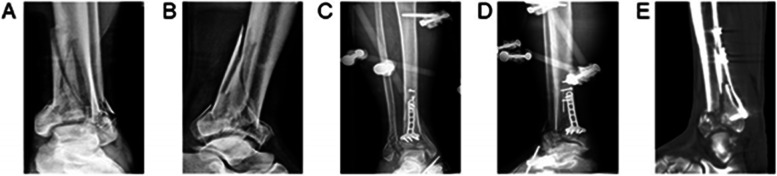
A 52-year-old man involved in a motor vehicle accident developed AO/OTA C3 pilon fracture. A & B) Preoperative anteroposterior and lateral radiographs showed the fibular fracture and distal tibial articular surface multi-fragment. C & D) The anteroposterior and lateral view of the ankle showed external fixation was used to maintain the length and axial alignment of the limb undergoing external and internal fixation in the first stage. E) The postoperative sagittal computed tomography scans showed that the congruency of the articular surface was not satisfactory after undergoing external and internal fixation in the first stage
